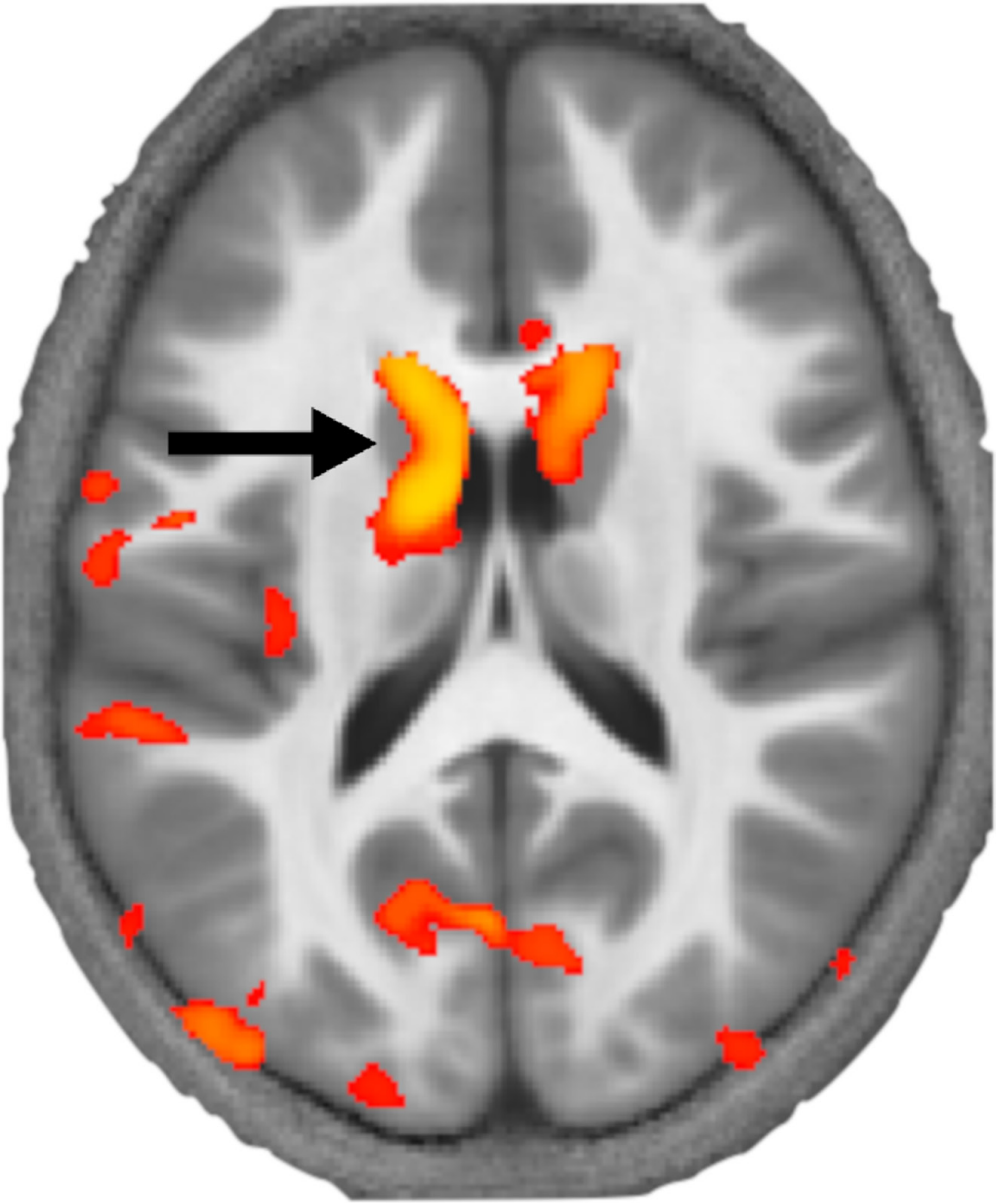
How levels of myelin differ in the brains of children with developmental language disorder. Krishnan et al. used a MRI technique called MTsat to image the brains of children with and without developmental language disorder. This image shows a section through the brain at the level of the basal ganglia with a coloured map (orange and yellow) overlaid representing the areas of the brain where myelin levels are reduced. This revealed that myelin levels are particularly low in a part of the striatum called the left caudate (black arrow) in children with developmental language disorder.
